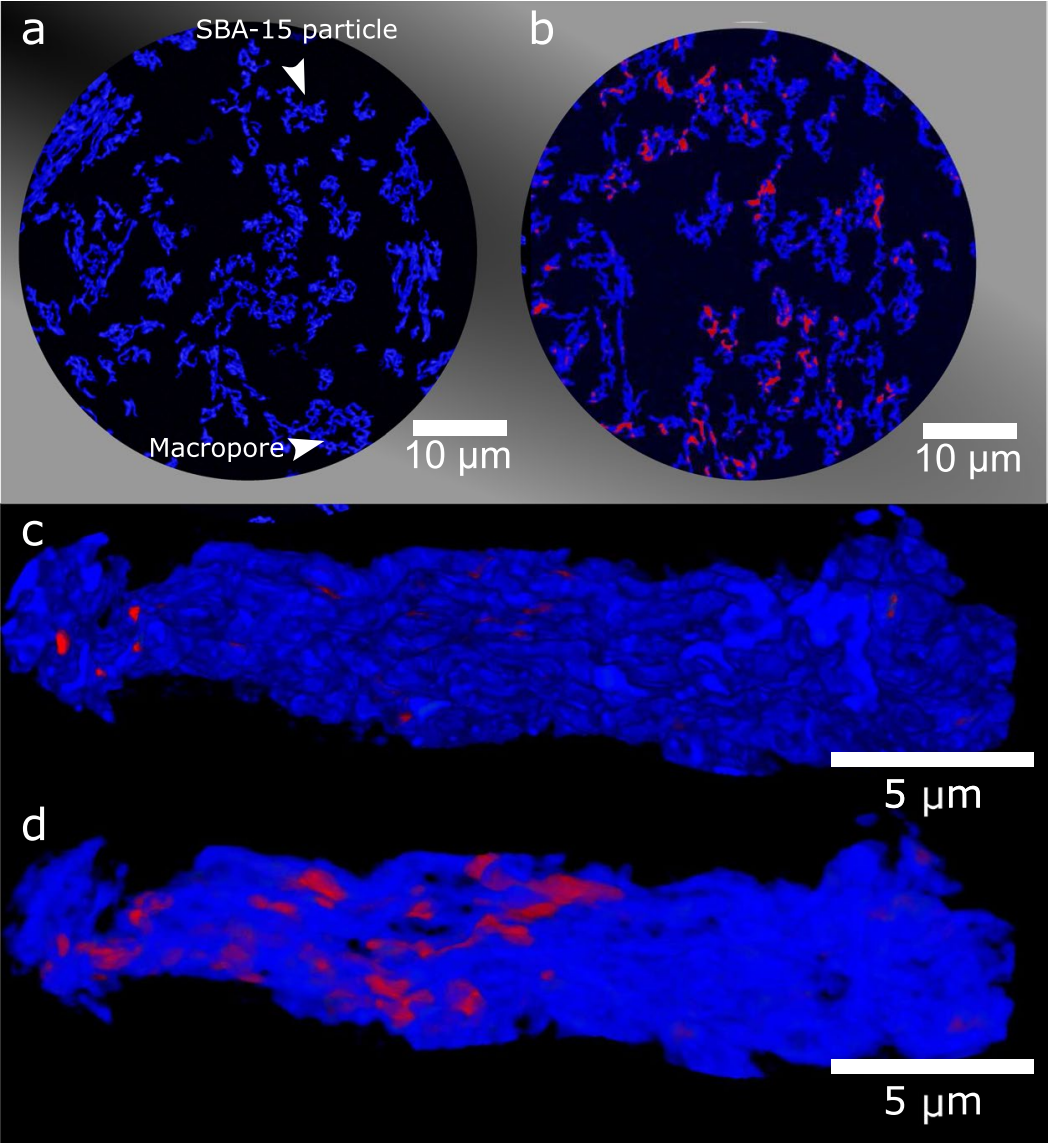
(a) X-ray tomogram, obtained at ID16B, of SBA-15, showing the macropores in the SBA-15 particles. (b) X-ray tomogram of SBA-15 with encapsulated HBsAg shown to agglomerate in the macropores. (c) 3D visualization of SBA-15 with HBsAg, showing small amounts of HBsAg at the surface, (d) Same particle as in (c), shown as semitransparent allowing visualising the agglomerated protein inside the macropores.
